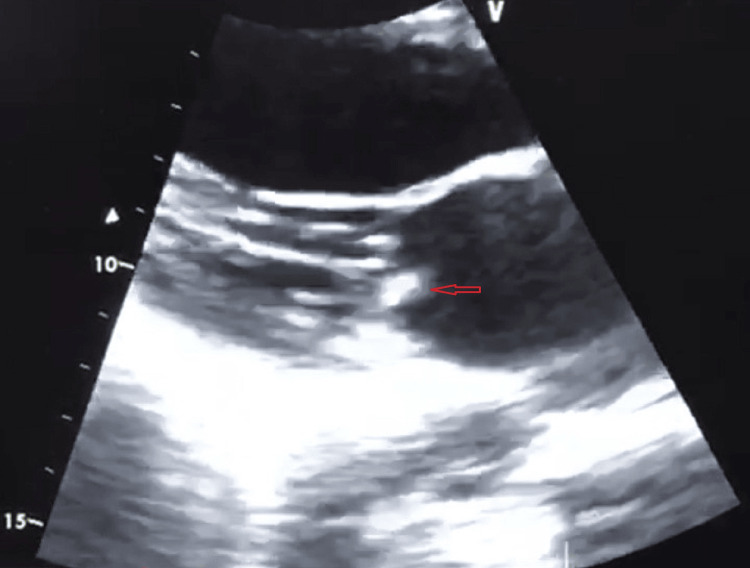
Transthoracic parasternal long-axis view. The image shows vegetations on the auricular side of the small mitral valve (arrow).
